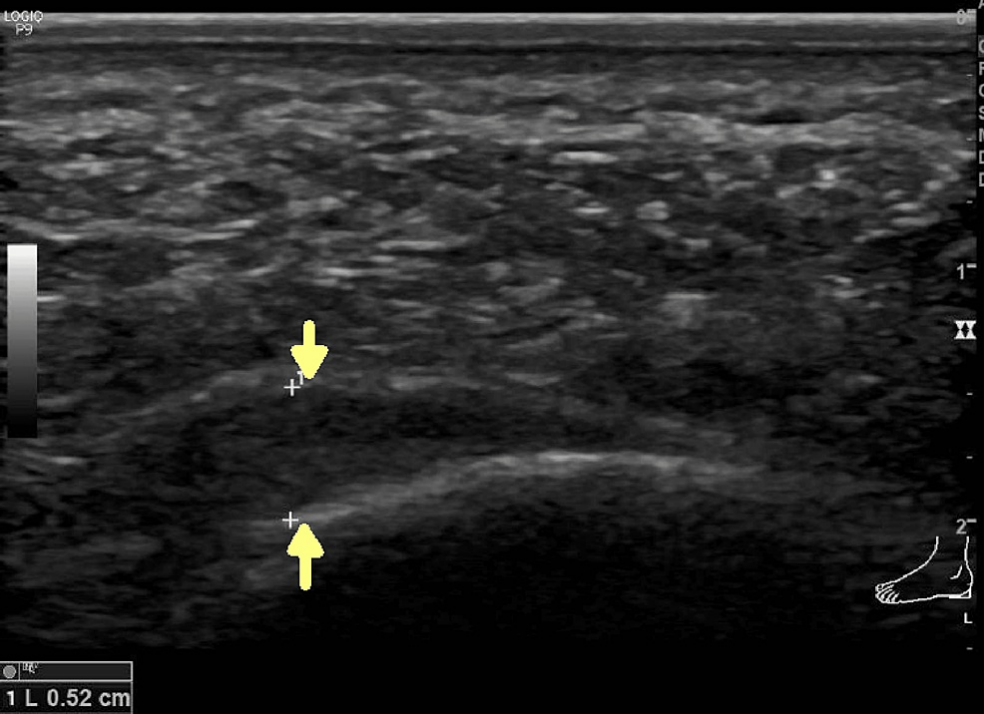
Ultrasonographic picture showing the thickened and hypoechoic plantar fascia
